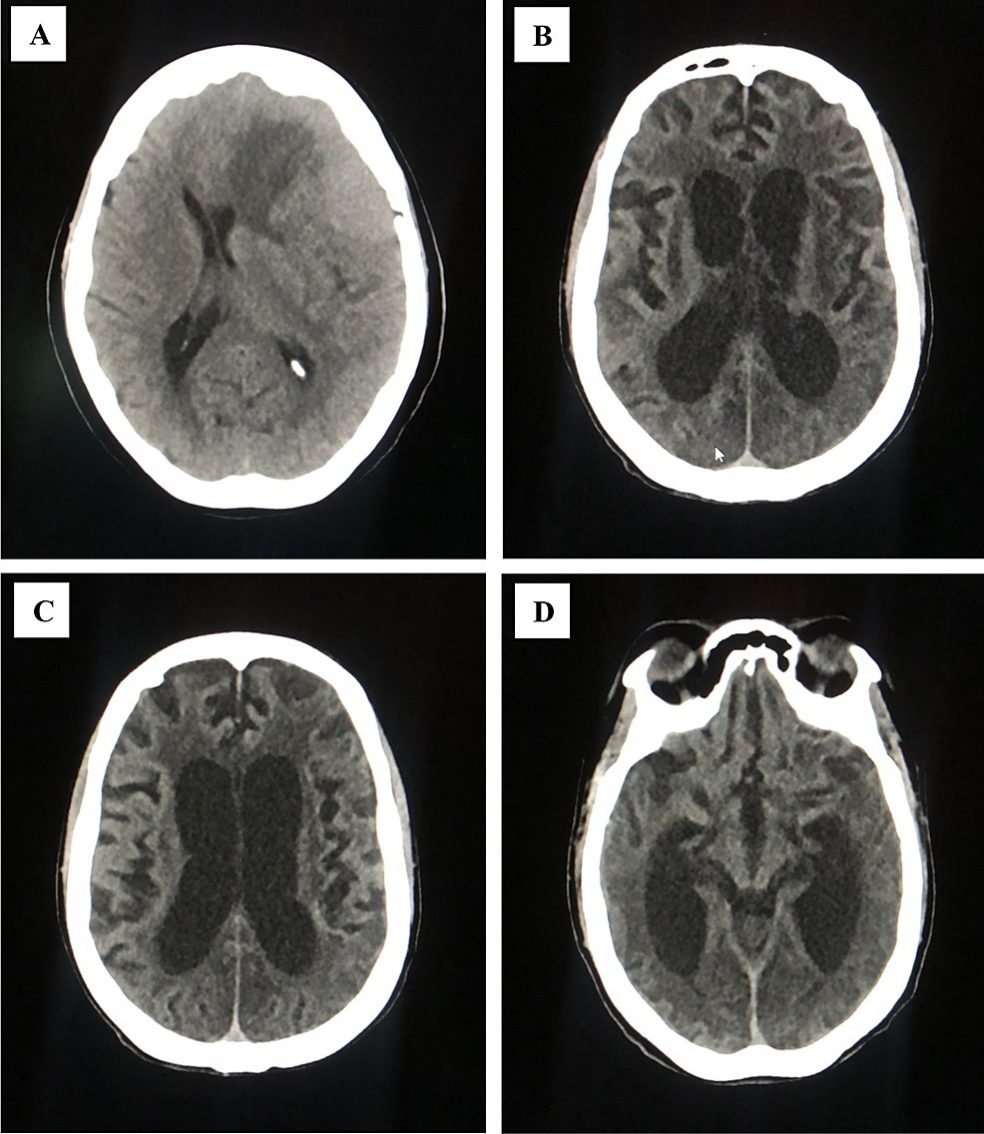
Non-contract CT scan of the brain of two patients with COVID-19 who had acute cerebrovascular complications (A) Large mass lesion. (B) Bilateral severe atrophic changes with diffuse extensive white matter hypodensities. (C) Loss of gray/white matter differentiation in the left parietal region. (D) Bilateral occipitotemporal loss of gray/white matter differentiation CT: computed tomography; COVID-19: coronavirus disease 2019
